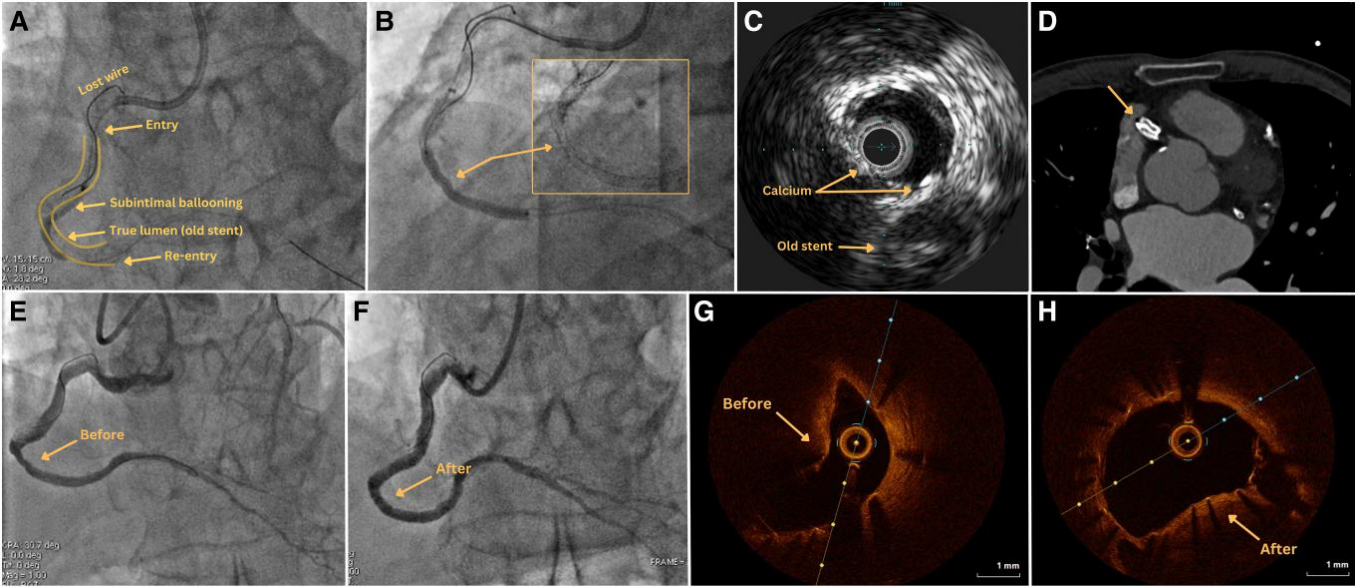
(*A*) Long, subintimal tracking outside the old stent during antegrade wiring. (*B*) Stent under-expansion in the subintimal segment (arrows). (*C*) Intra-vascular ultrasound confirms the intra-stent calcified chronic total occlusion and calcifications in the media. (*D*) ‘Double-barrel’ appearance of the two stents, computed tomography (arrow shows two parallel stents, one occluded situated in the ‘old true lumen’ and one subintimal, now realizing the permeable lumen). (*E*, *F*) Before and after intravascular lithotripsy of the subintimally located stent. (*G*, *H*) Optical coherence tomography before and after intravascular lithotripsy.
